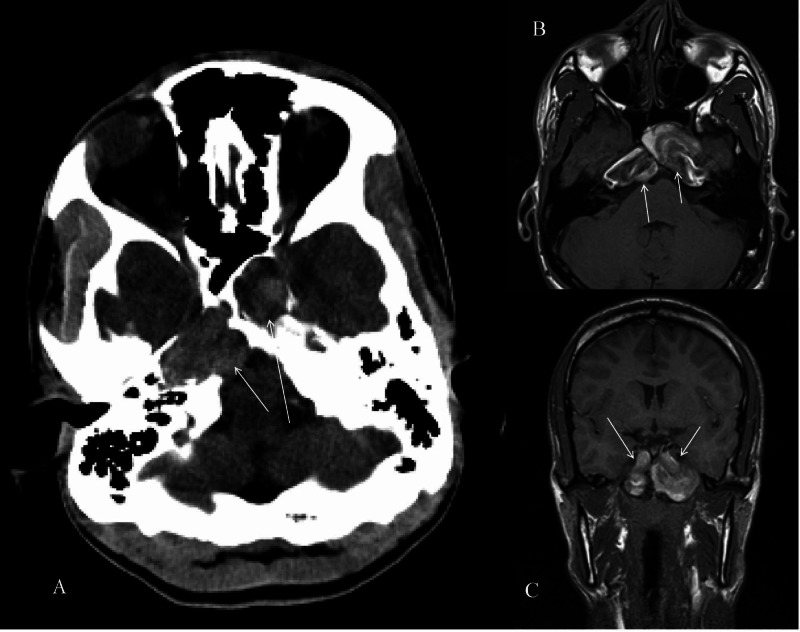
CT transverse (A), MRI transverse (B), and MRI coronal (C) revealed bilateral lesions of the petrous segment of the internal carotid artery (ICA) bilaterally
